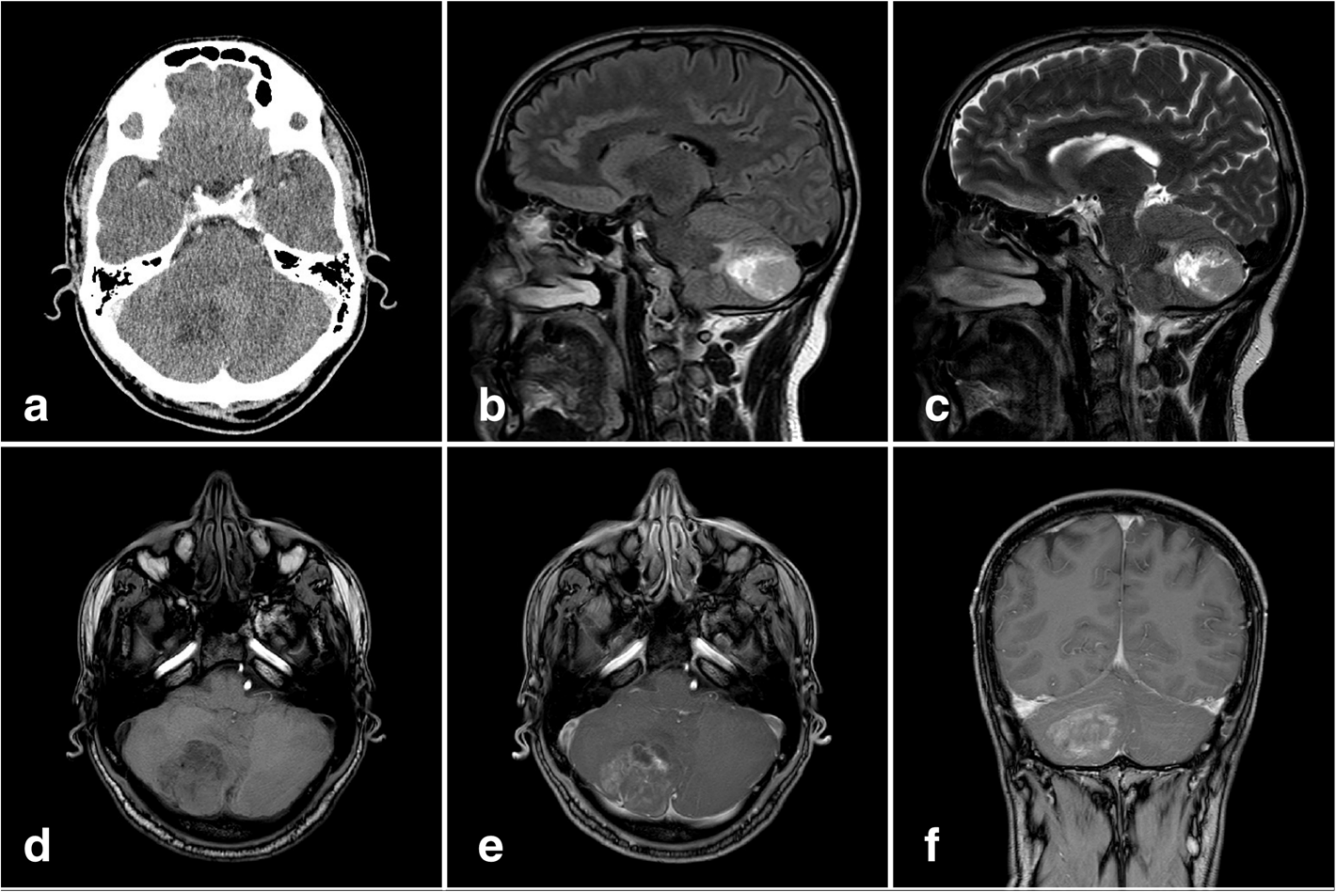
Computed tomography shows a hypointense ill-defined lesion of the right cerebellar hemisphere (a). The lesion presents a hyperintensity on fluid-attenuated inversion recovery sequence (b) and T2-weighted imaging (c). On T1-weighted imaging, the lesion shows a hypointensity (d). A light, diffuse contrast enhancement can be observed on T1-weighted imaging with contrast (e + f)
